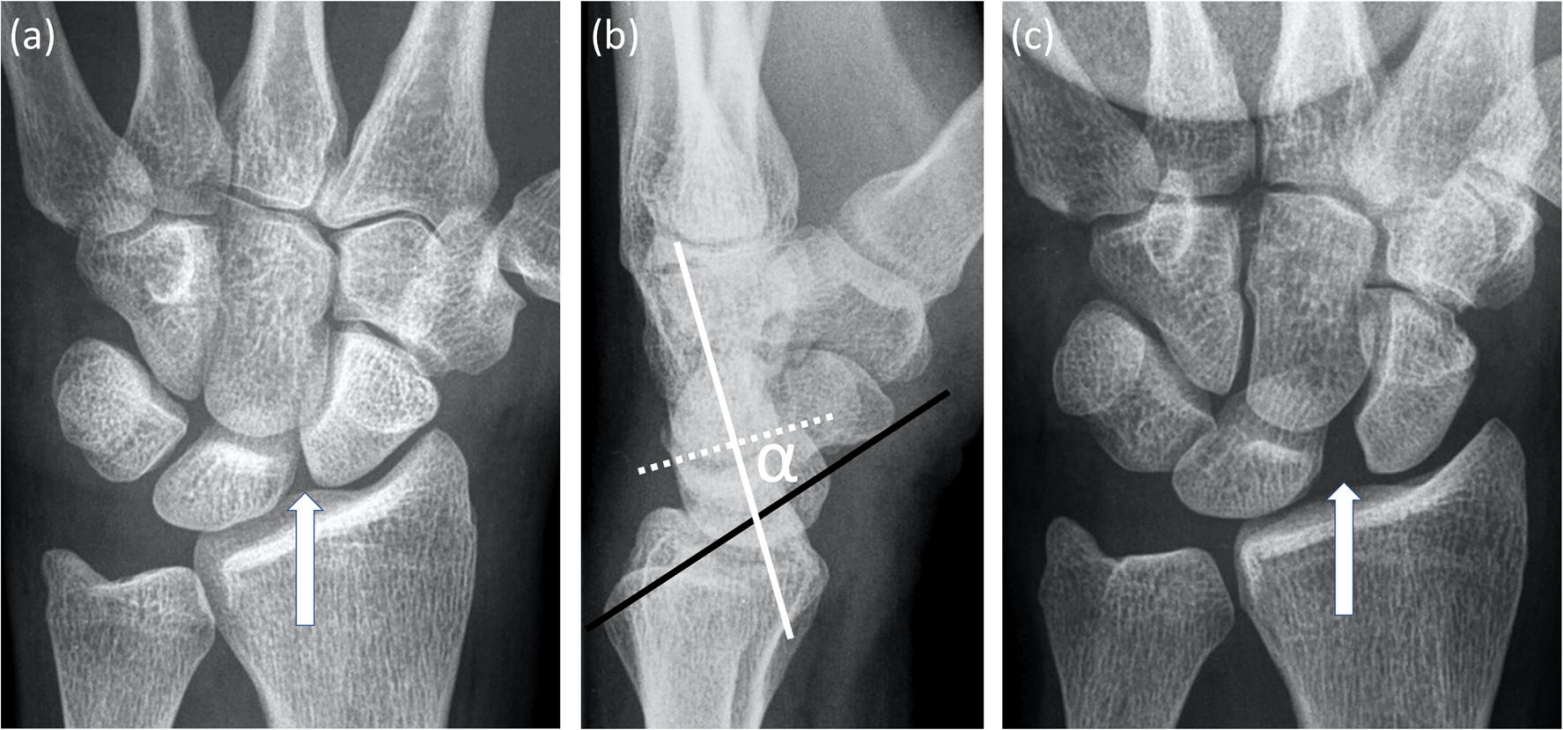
A 28-year-old male patient with symptoms of scapholunate joint instability after a left-sided rotational wrist injury due to accidentally jammed drilling machine. a Dorsopalmar radiograph shows a slightly increased scapholunate distance (arrow) and a signet ring sign of the scaphoid. b The lateral radiograph presents an abnormally increased scapholunate angle of 73° (α). c Dorsopalmar clenched ball view as a radiographic stress view demonstrates a definitely abnormal increased scapholunate distance
